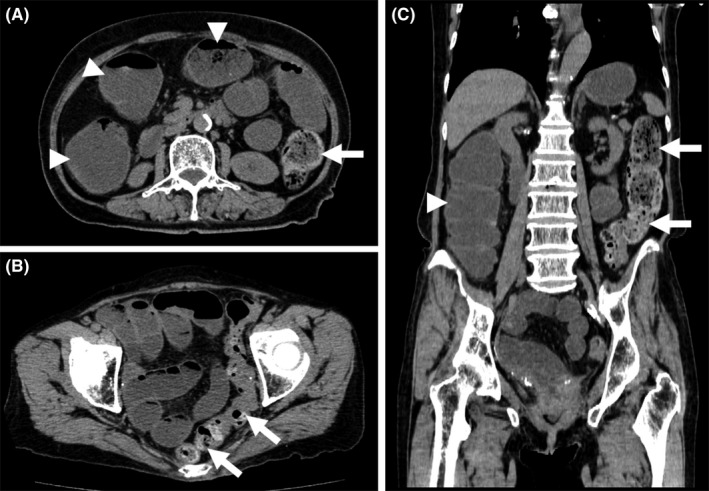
Computed tomography. Axial (A, B) and coronal (C) views show fecal impaction in the left colon and rectum (arrows) and dilatation of the oral side of the colon (arrowheads)
